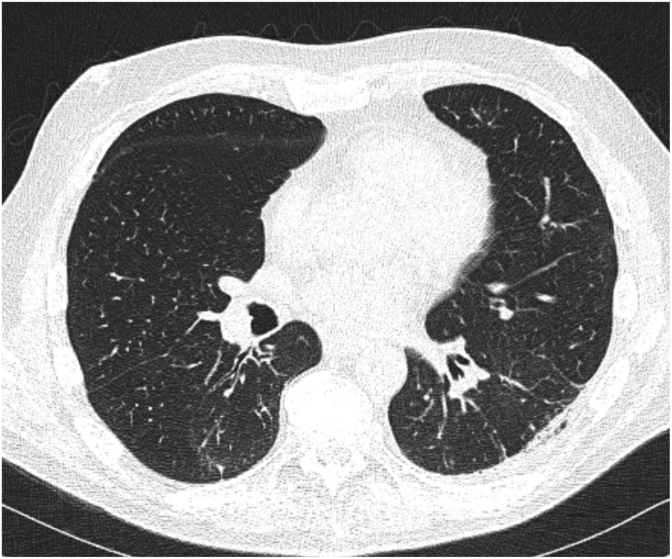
Follow up CT scan of the abdomen at Day+77 (11 weeks) showing complete resolution of previously bilateral pulmonary nodules.
